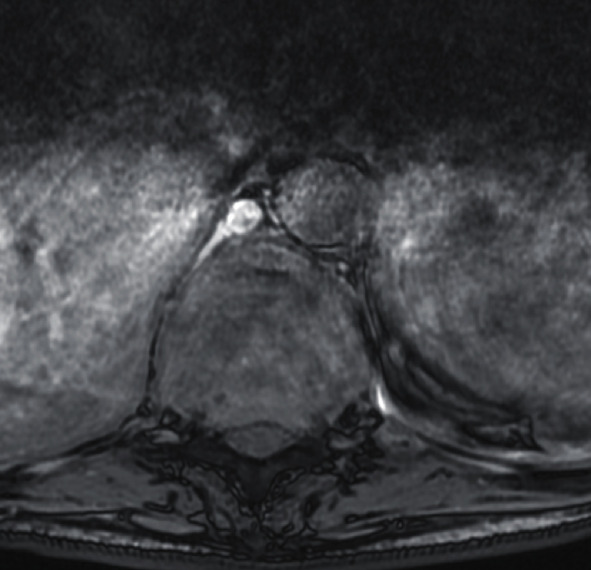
Preoperative MRI (axial image, level of compression).
